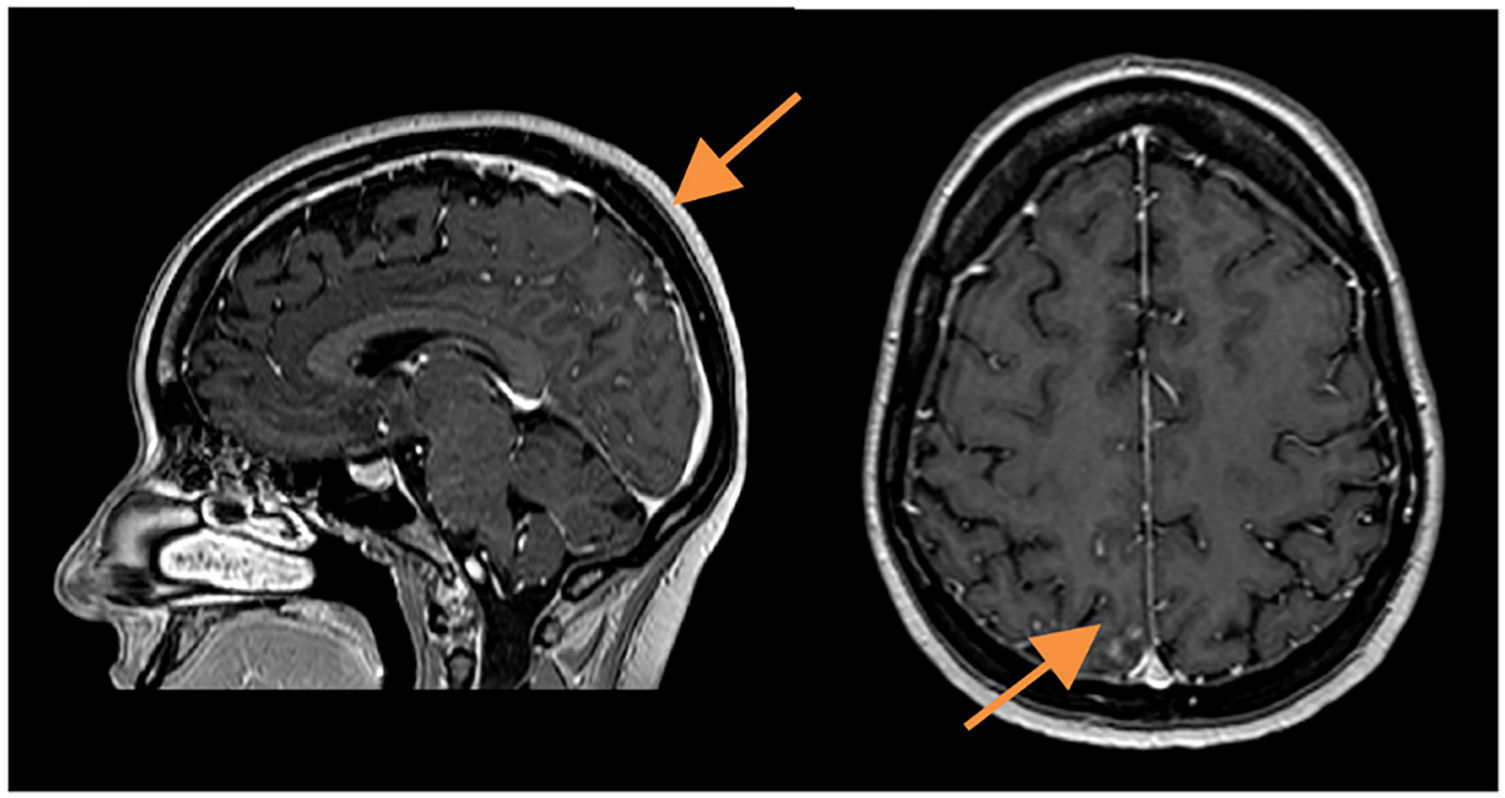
CE MRI response evaluation after 3 cycles of T-DXd. Right parietal lesion was residual and pachymeningeal infiltration was not found (arrows).
